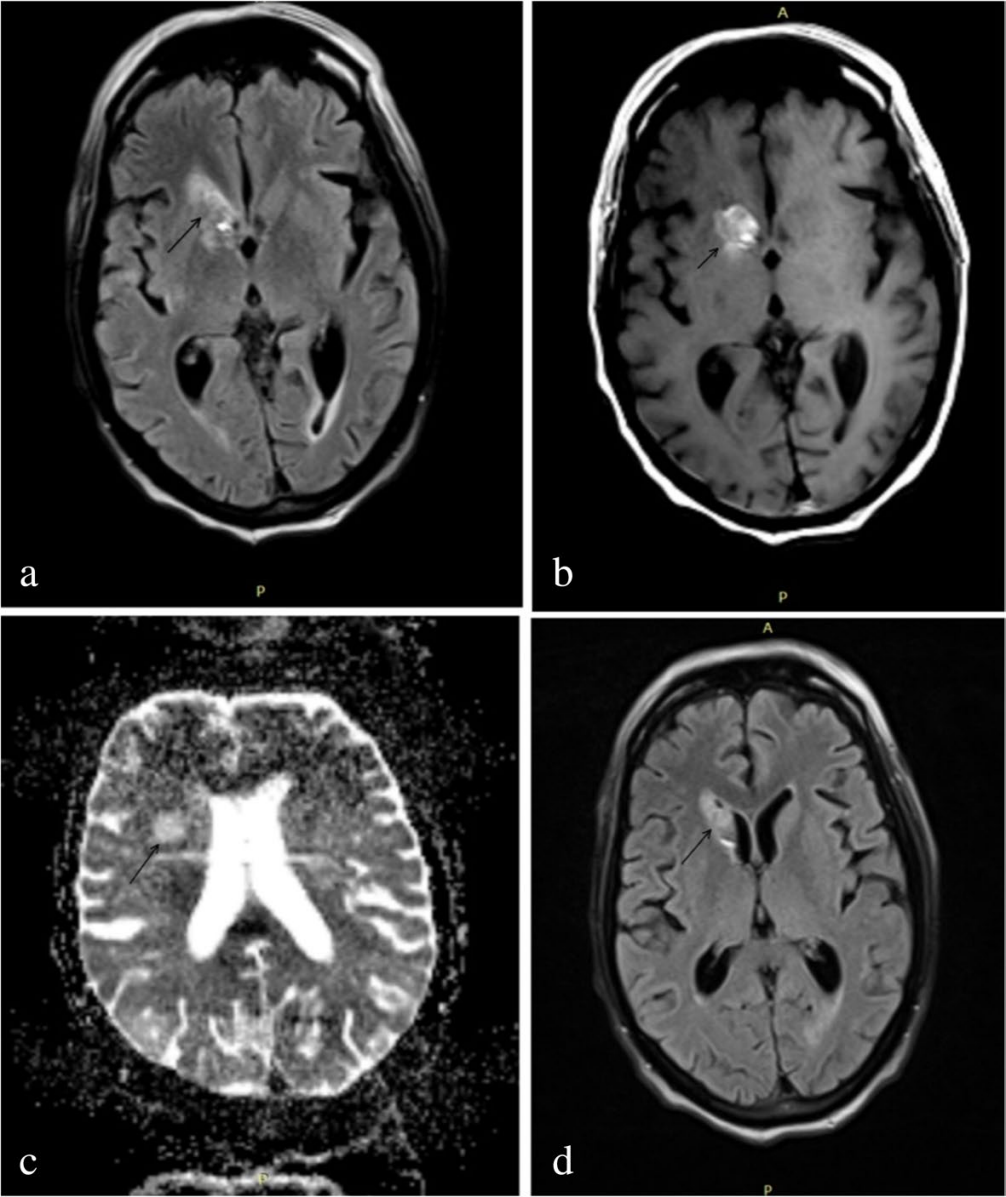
MRI of the brain showing a hyperintense lesion involving the right caudate nucleus on FLAIR (a, b), diffusion-weighted (c) and non-contrast T1-weighted (d) axial images suggestive of an infarct with haemorrhagic transformation or haemorrhagic metastais to the brain
